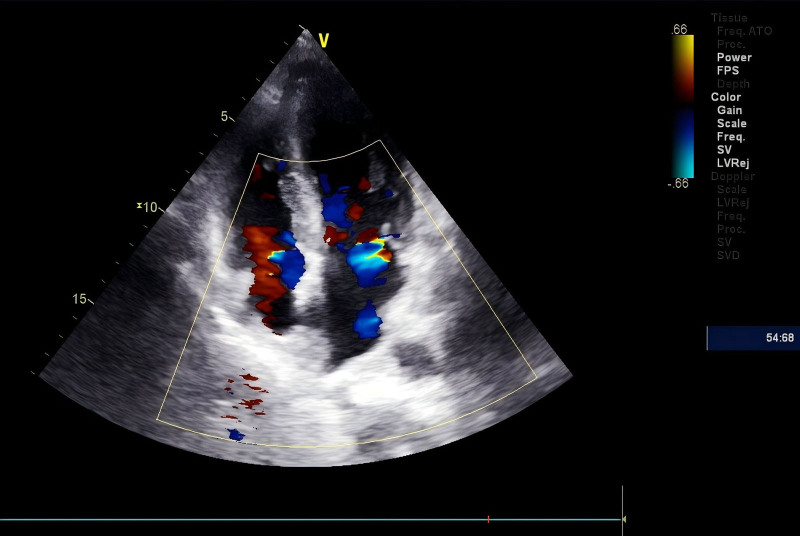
Color Doppler ultrasonography showed that the left ventricular wall was thickened, diastolic function decreased, both left and right atria enlarged, and atrial septum thickened.
